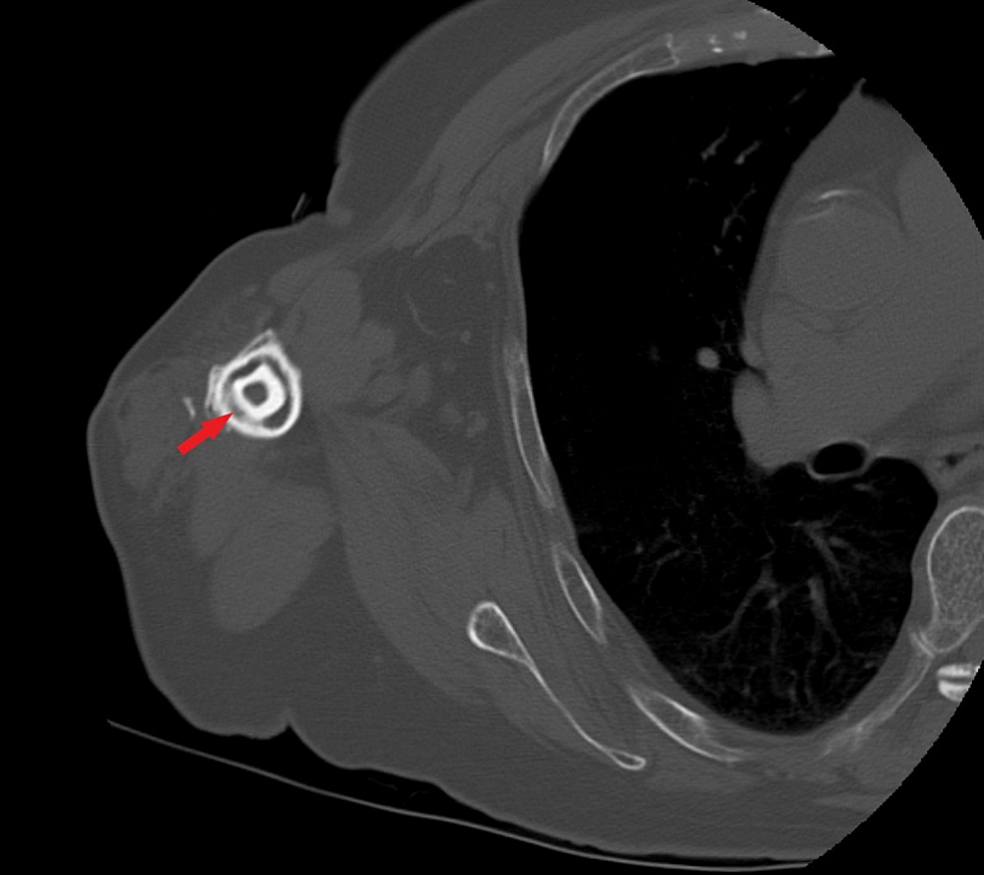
Axial cut of CT scan of the proximal humerus demonstrating osseous integration of fibular strut into the humeral canal (red arrow).
